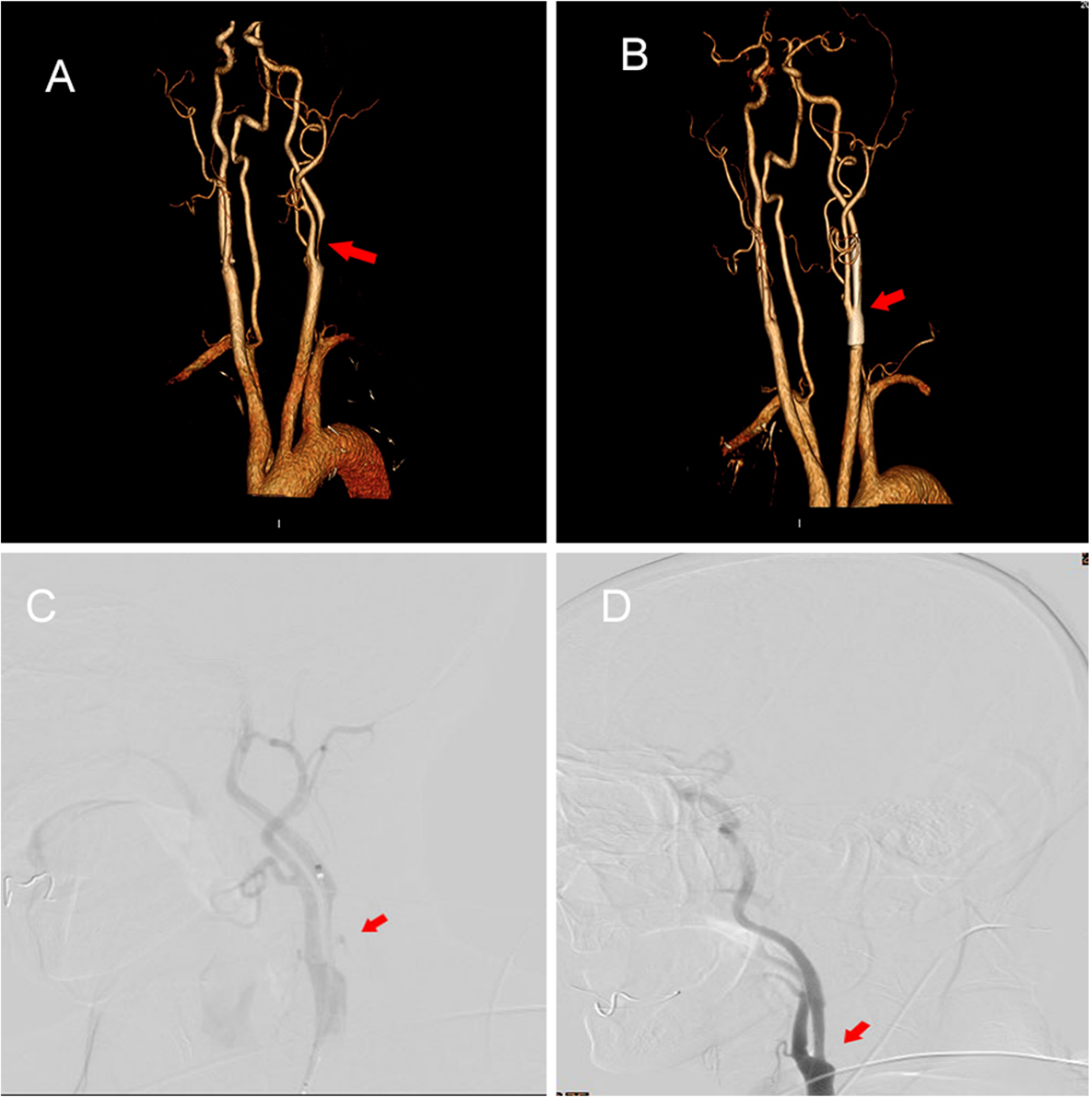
Internal carotid artery of the patient. a: Severe left carotid artery stenosis in the neck CTA. b Left carotid artery lumen unobstructed after CAS in the neck CTA. c Severe left internal carotid artery stenosis in the DSA. d Stent implantation lumen unobstructed in the DSA. DSA: digital subtraction angiography
